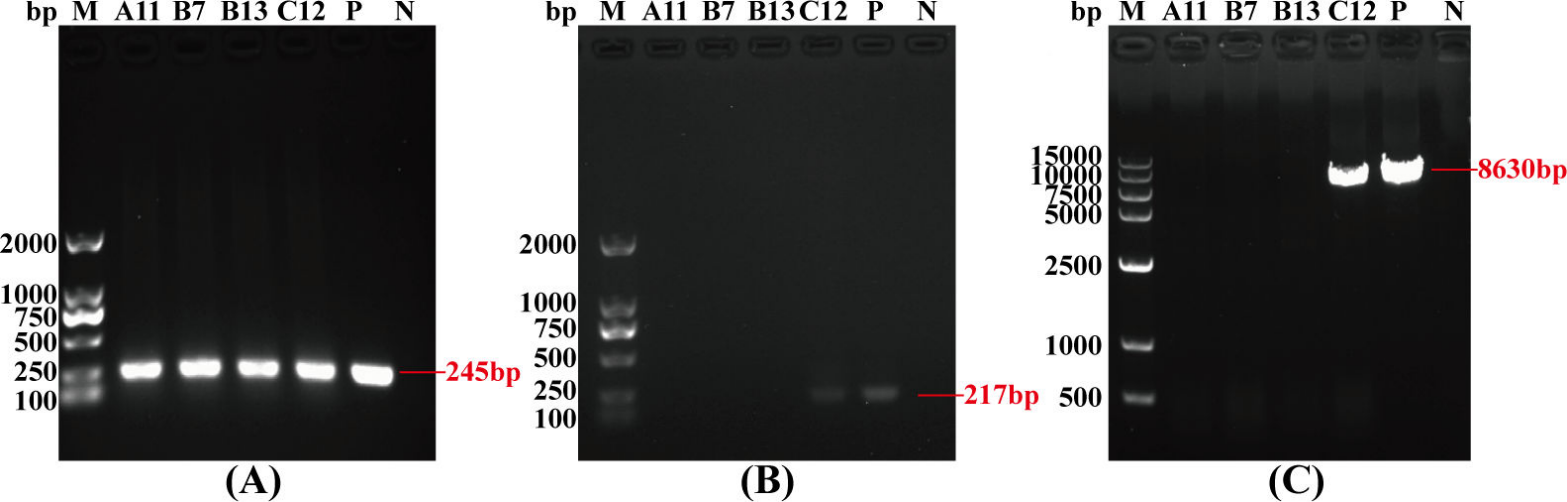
PCR-based agarose gel electrophoresis screening of positive clonal cells. (A–C) Screening and confirmation of four positive clonal cell lines using CT-F1/R1, CT-F2/R2, and CT-F3/R3 primers. gDNA was extracted from monoclonal cells for PCR analysis. Primer pairs CT-F1/R1, CT-F2/R2, and CT-F3/R3 amplified the head, tail, and approximate full length (245, 217, and 8,630 bp) of the CT insertion sequences, respectively. The amplification products were analyzed by electrophoresis on 1% agarose gel. The sizes of the amplicons were as expected, indicating the successful integration of the corresponding portions of the target insertion sequences into the genome. Lane M: 2,000- or 15,000-bp DNA marker; Lane N: negative control (wild-type HEK293T cells); Lane P: positive control (the residual mixed pool cells after flow cytometric sorting).
